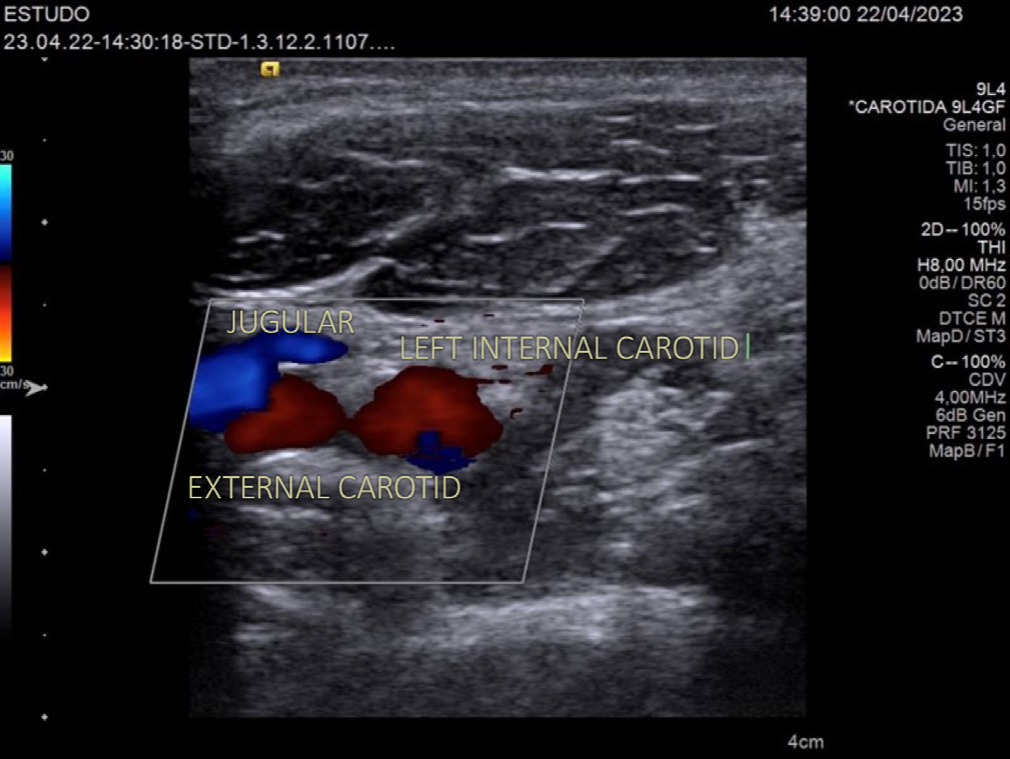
Transverse color Doppler flow ultrasound image showing the left internal carotid and left external carotid arteries and the left jugular vein.
